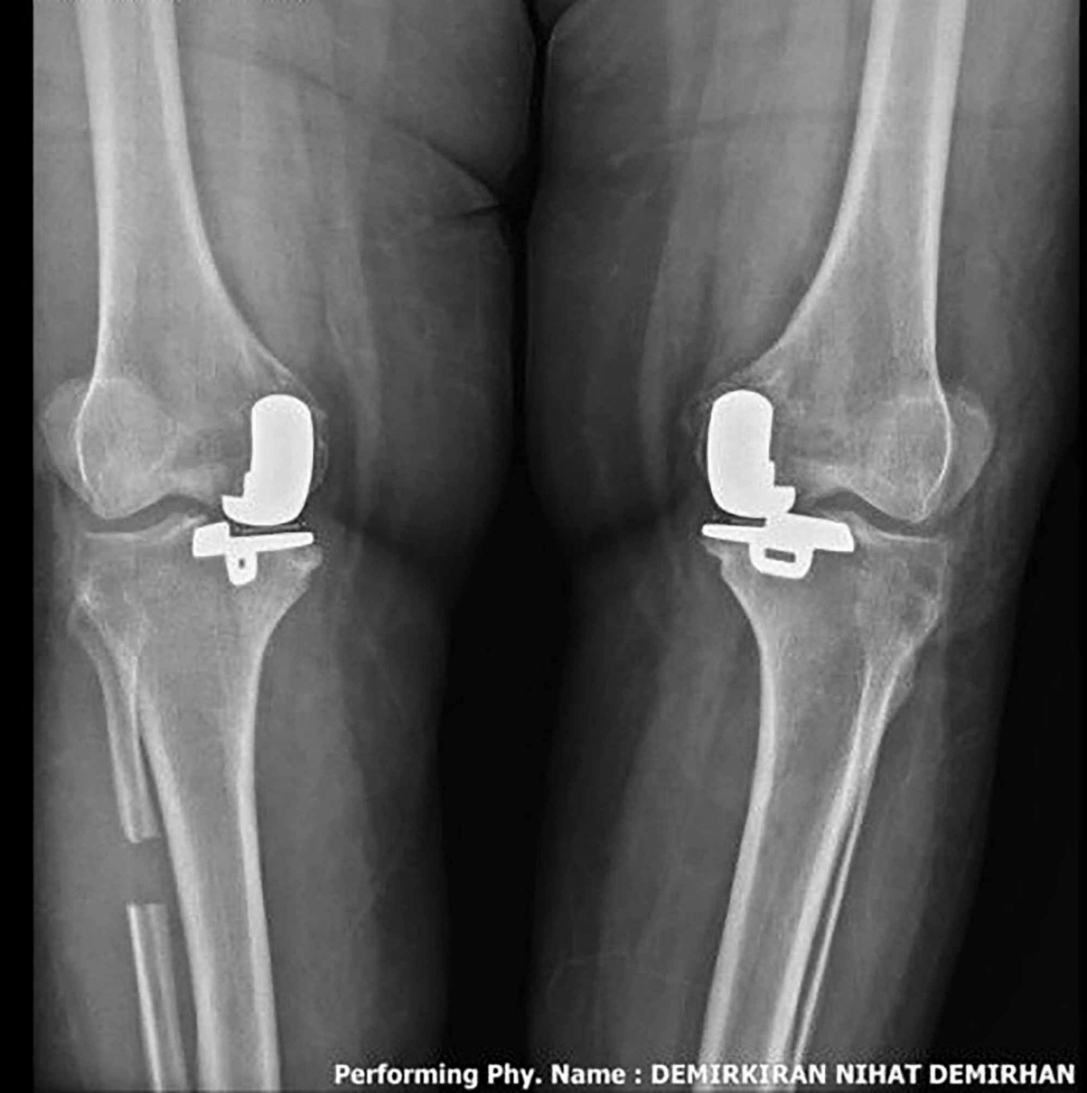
Full weight-bearing antero-posterior (AP) radiograph of both knees at six week follow-up
